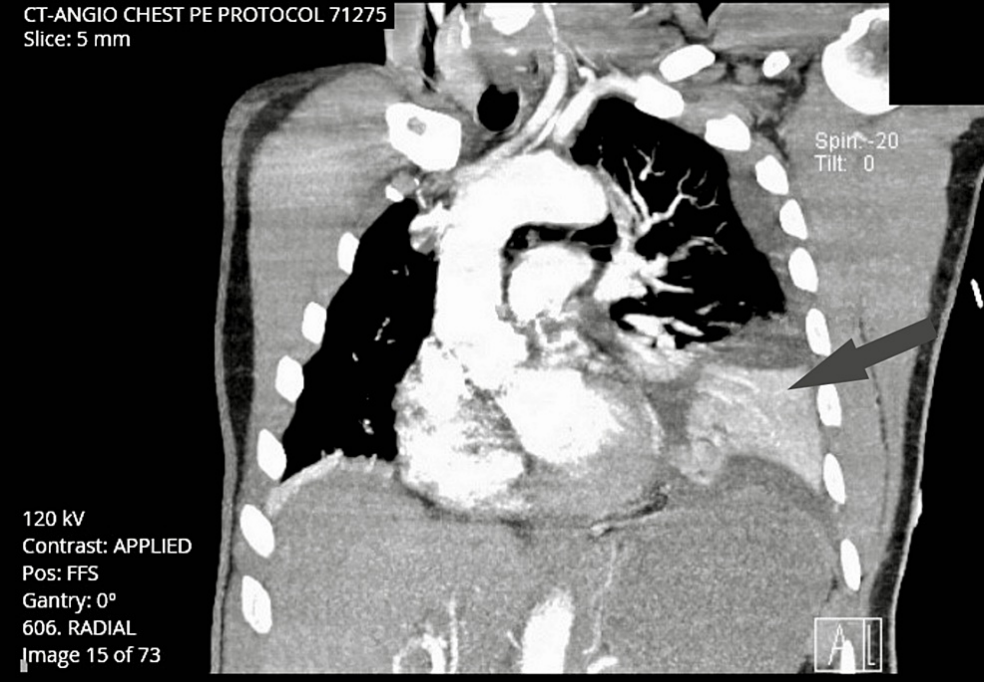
CT angiogram showing pleural effusion (arrow) PE: pulmonary embolism; FFS: feet first-supine.
